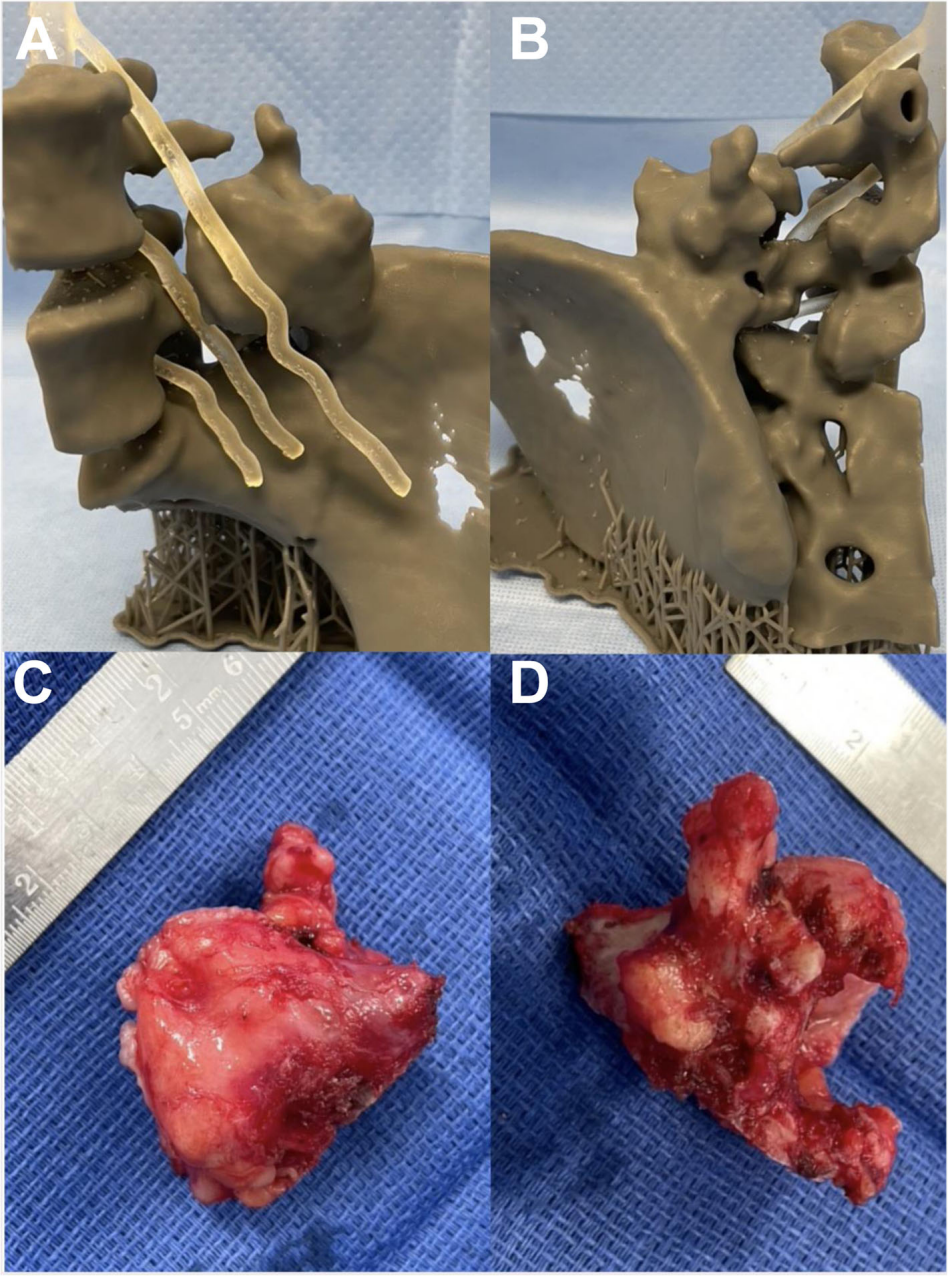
3D-printed model combining the CT and the MRI elements demonstrating the exact proximity of the nerve roots to the tumour. Anterior (A) and posterior (B) views. Corresponding anterior (C) and posterior (D) views of the excised osteochondroma
